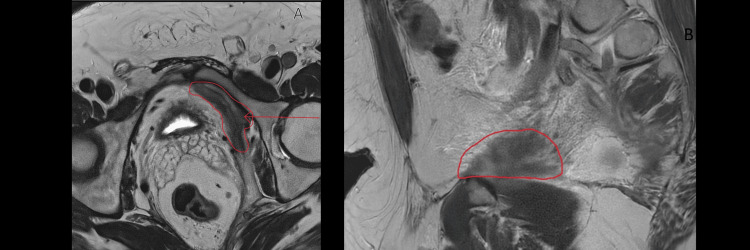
MRI Pelvis (January 2025) This scan identified new, multifocal disease. (A) Axial T2-weighted view shows the new left pelvic sidewall mass (outlined in red). (B) Sagittal T2-weighted view shows the associated abnormal thickening (outlined in red).
